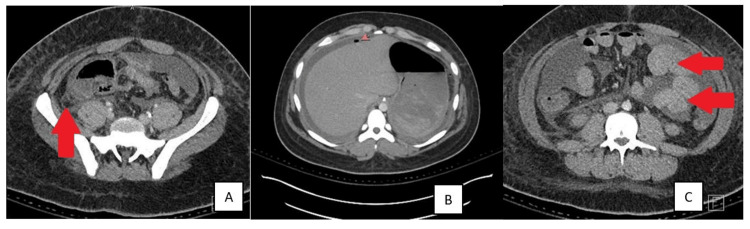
A computed tomography scan of the abdomen and pelvis demonstrated perforated appendicitis [A] with pneumoperitoneum [B] and multiple intra-abdominal fluid collections [C], the largest of which was located in the right upper quadrant.
